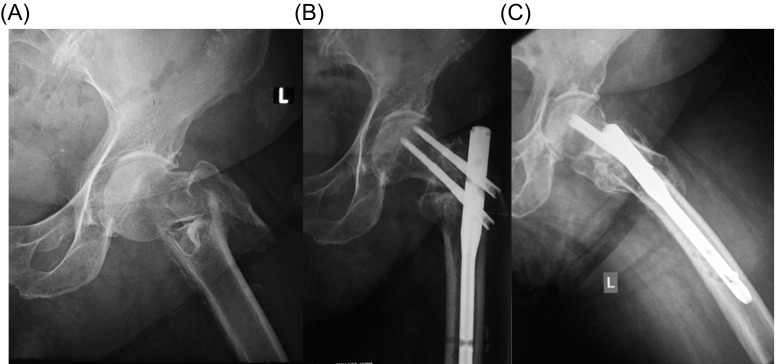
(A) Anteroposterior radiograph of the left hip of an 81-year-old woman shows an AO/OTA-31-A3 fracture. (B) Anteroposterior and (C) lateral radiographs of the left hip one year after surgical treatment show fracture healing. The patient was alive with good function.
